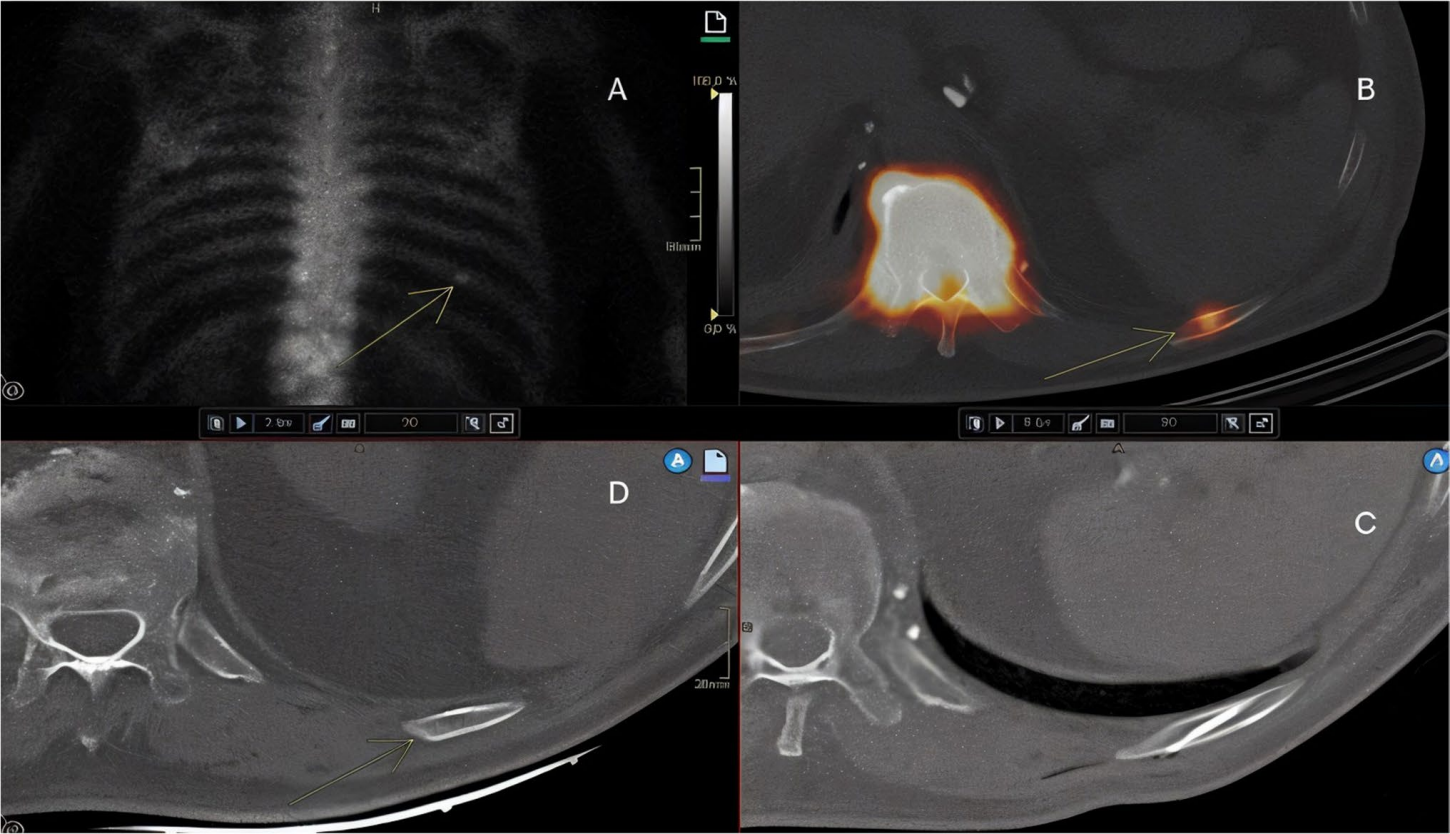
A 73-year-old patient with newly diagnosed prostate cancer. Gleason-7. Skeletal scintigraphy shows high uptake in the left 10th rib with slightly sclerotic lesion in the same place on CT-examination. Bone biopsy shows hemangioma. No metastasis
